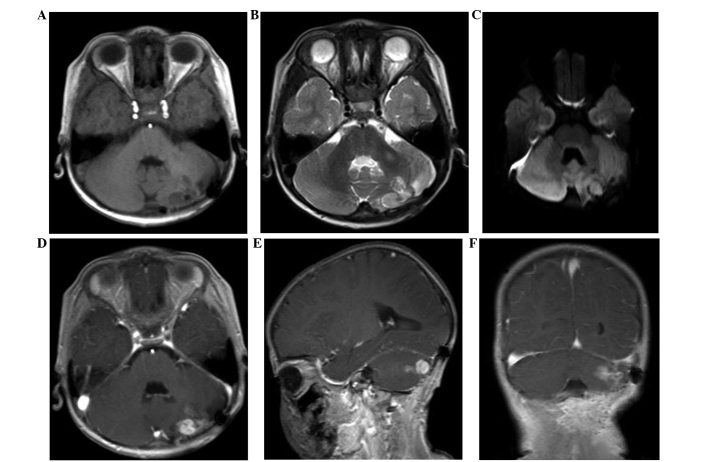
Magnetic resonance imaging of the brain one month after surgery. (A) T1WI, (B) T2WI, (C) diffusion-WI and (D-F) T1WI with gadolinium suggesting that a small relapsing tumor had occurred in the same region. WI, weighted imaging.
